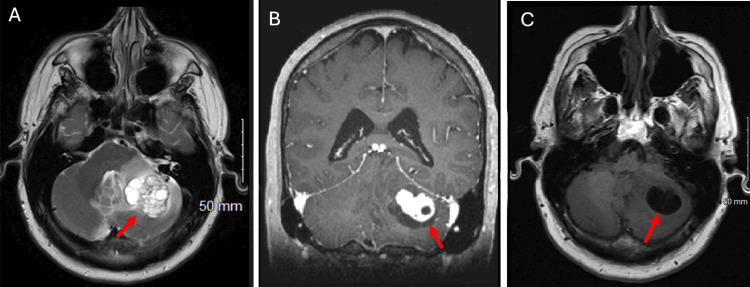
The patient's preoperative magnetic resonance imaging (MRI) T2-weighted axial MRI brain, post contrast showing a large heterogenous mass lesion in left cerebellum (panel A, red arrow) with intensely enhancing peripheral nodules (diameter 33 mm x 34 mm x 27 mm). T1-weighted coronal, post-contrast MRI brain demonstrating extensive vasogenic edema (panel B, red arrow) that extends to the vermis and displacement of cerebellar tonsils. T1-weighted axial, post-contrast MRI brain showing hypointense cystic lesion (panel C, red arrow) causing mass effect on the fourth ventricle and vermis.
